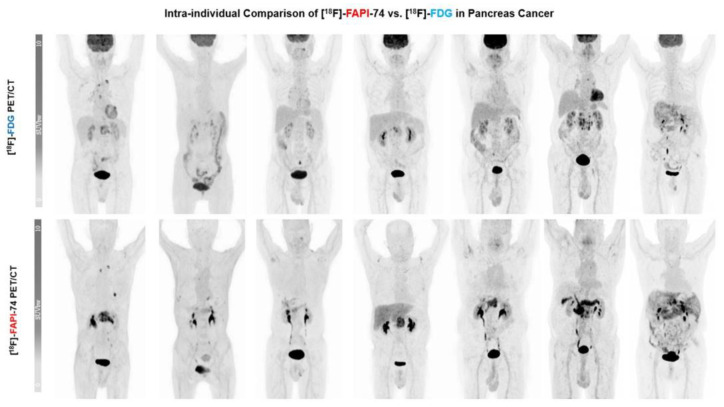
An overview of maximum intensity projections (MIP) in all seven patients, [^18^F]FDG vs. [^18^F]FAPI-74.
